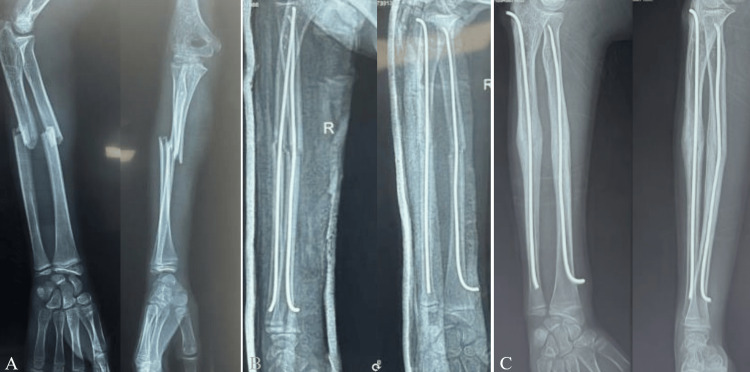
Ten-year-old boy with a displaced overlapping mid-shaft forearm fracture on the preoperative radiograph (A), postoperative radiograph showing intramedullary nails in both forearm bones (B), and radiograph obtained before nail removal demonstrating callus formation with good alignment (C) These images are owned by our patient.
